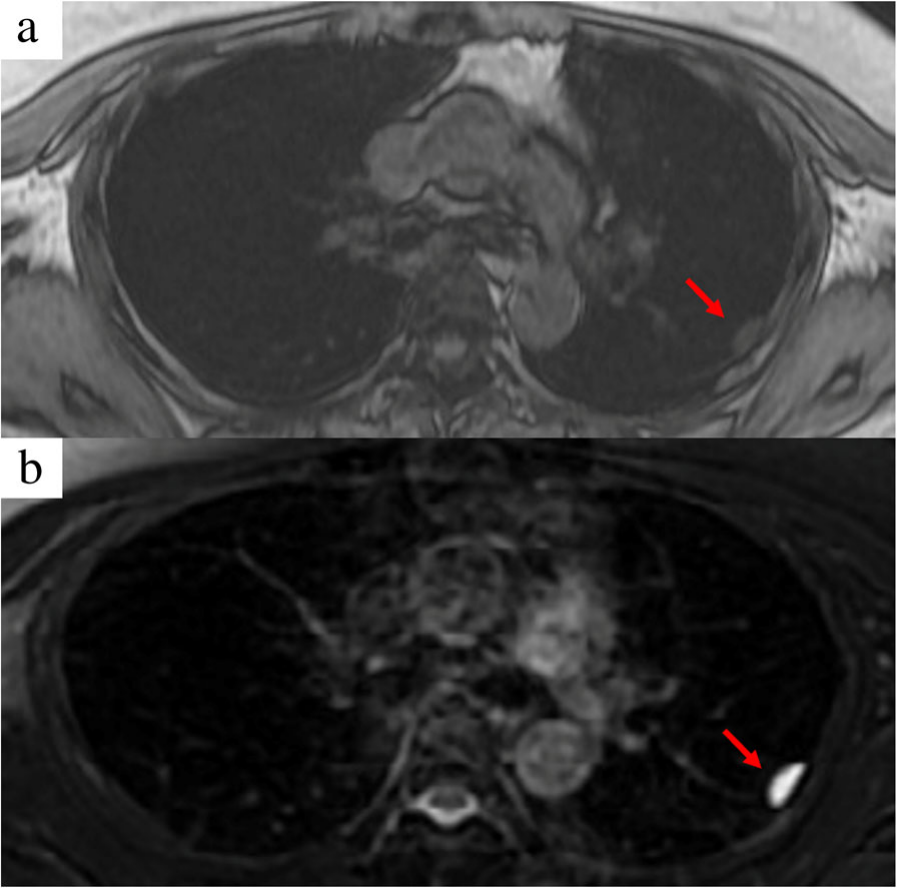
Magnetic resonance imaging demonstrated a low-intensity mass (*arrow*) in T1-weighted imaging (a) and high-intensity mass (*arrow*) in T2-weighted imaging (b)
